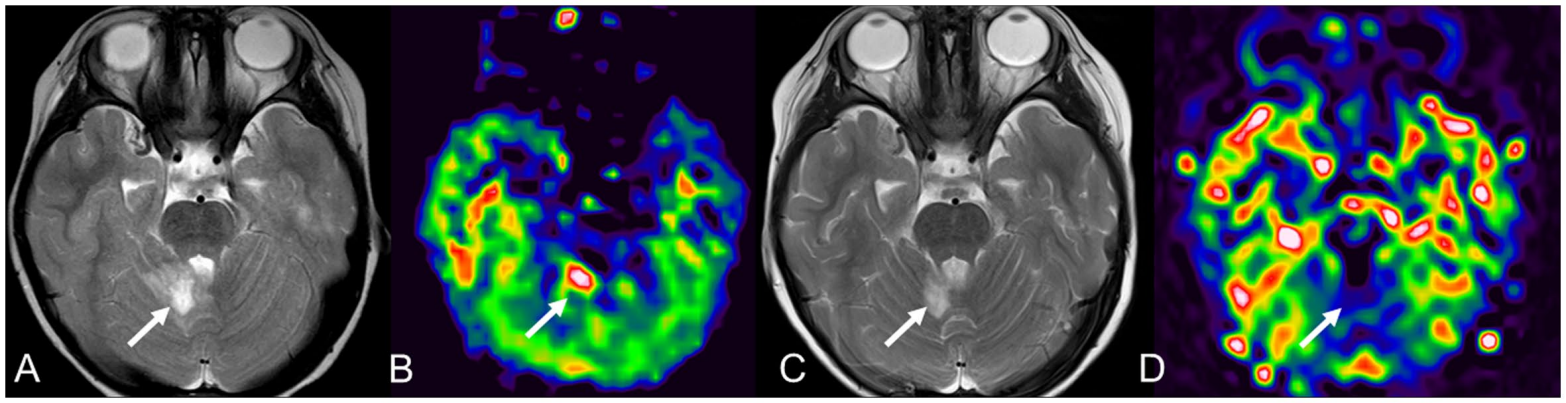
A 3-year-old female with cerebellitis associated with myelin oligodendrocyte glycoprotein-immunoglobulin G-associated disease without neurological sequelae. She has hyperintensity on T2WI (A, arrow) and hyperperfusion on ASL (B, arrow) in the right cerebellum on day 7. Follow-up MRI on day 18 shows a slight decrease in the area of hyperintensity on T2WI (C, arrow) and hypoperfusion on ASL (D, arrow). T2WI, T2-weighted imaging; ASL, arterial spin labeling; MRI, magnetic resonance imaging.
